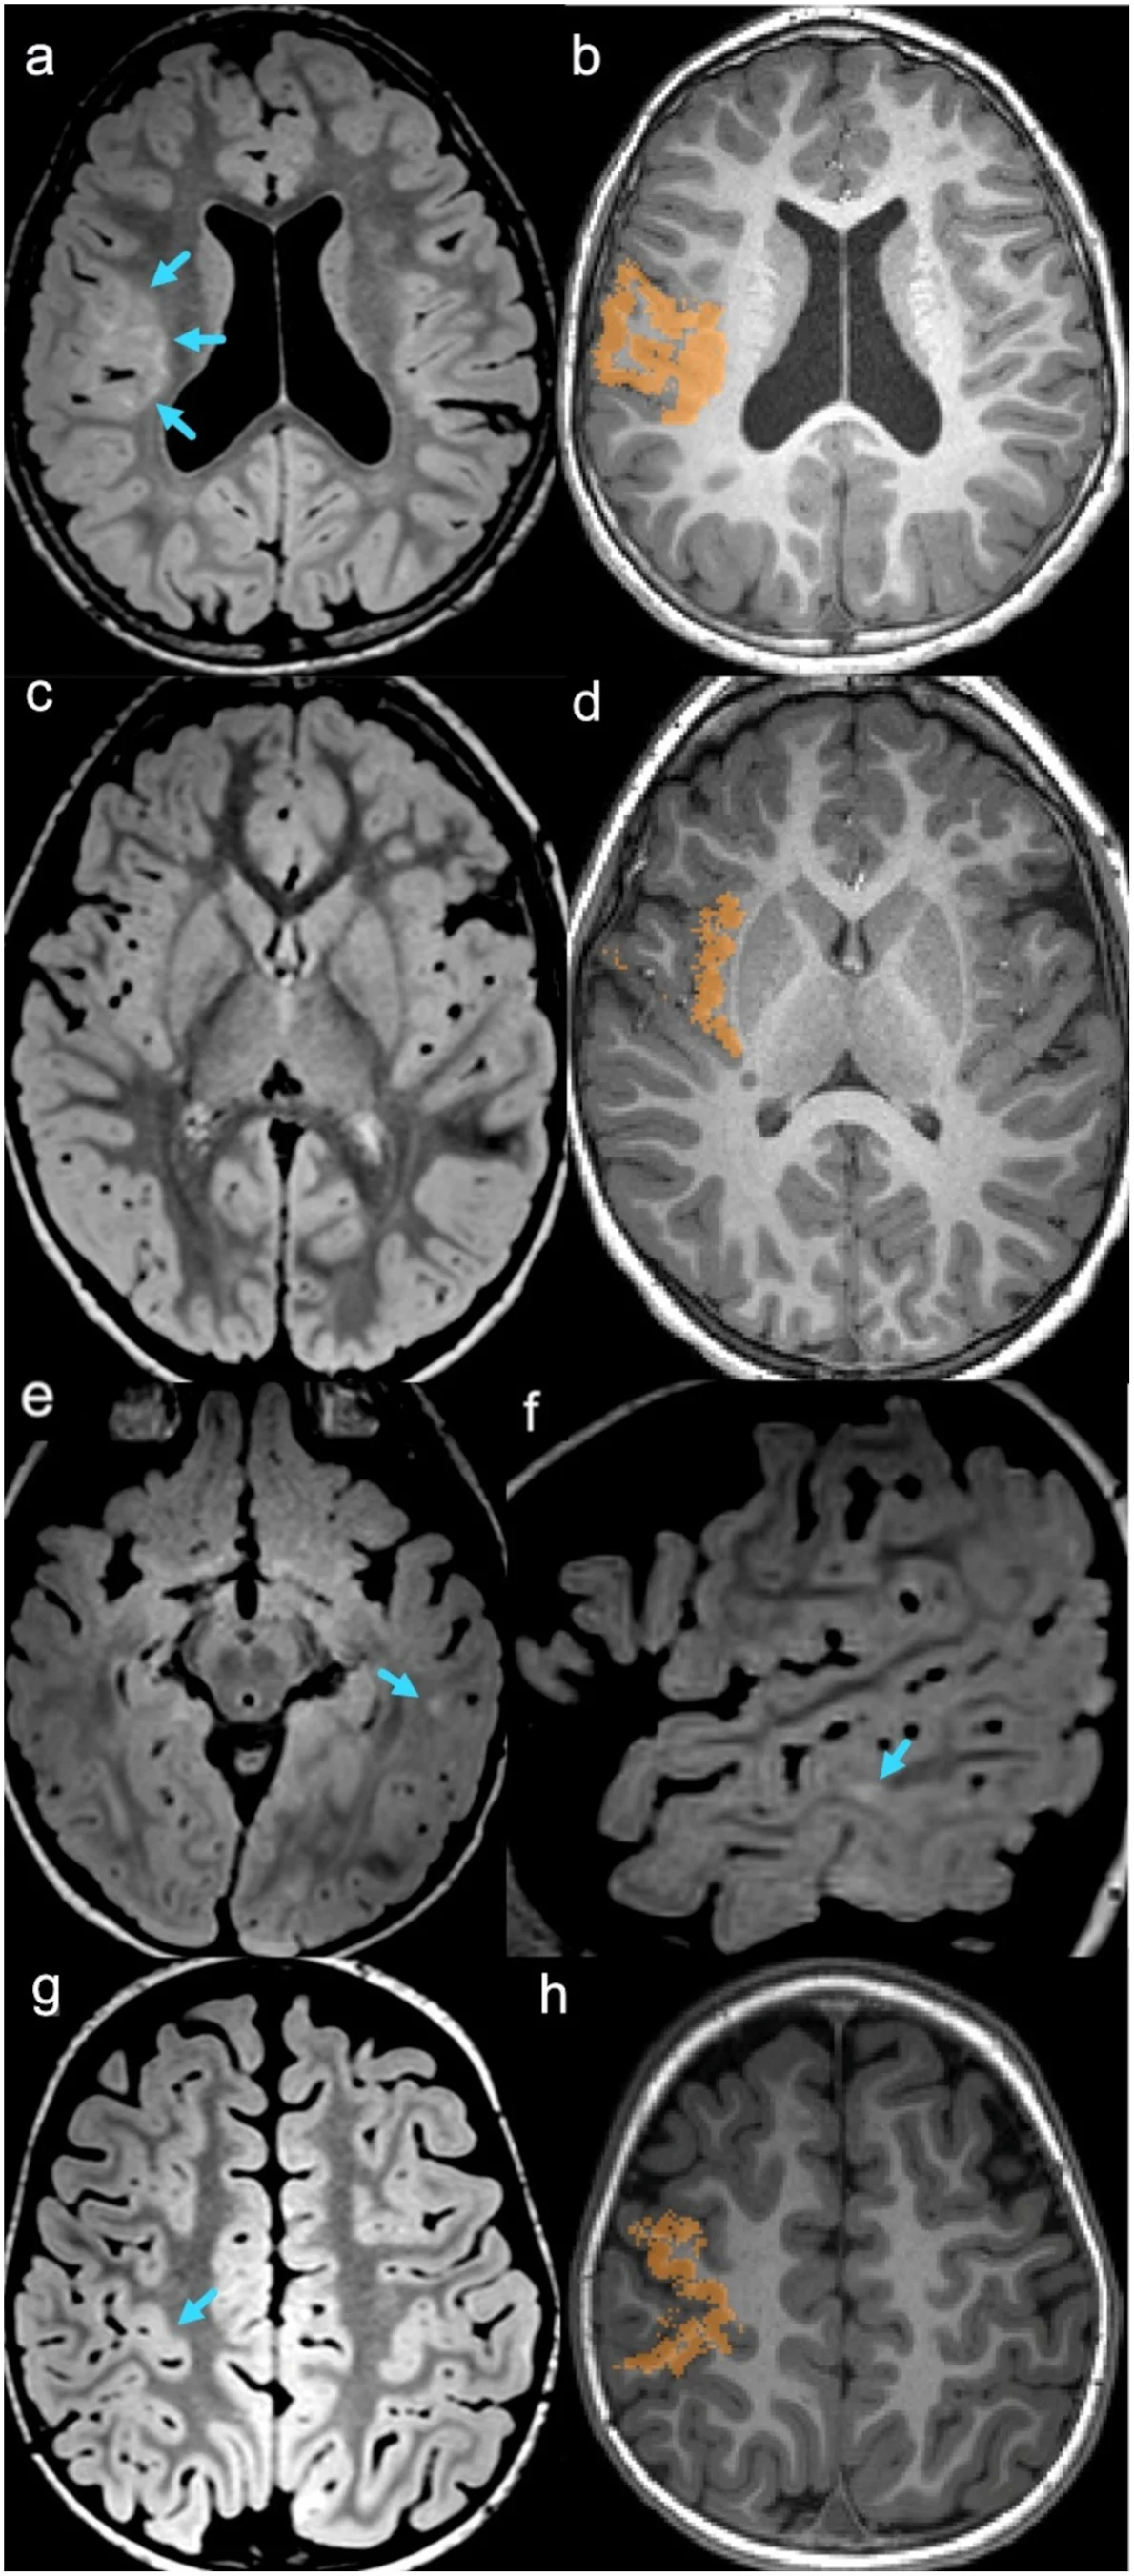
Representative imaging examples (MELD Graph): (a–b) Correctly identified lesion (TPL) in the right insula consistent with presumed FCD type 2A, shown on FLAIR (blue arrows, a) and the corresponding MELD Graph lesion mask on 3D T1-weighted MRI (b). (c–d) False-positive MELD Graph detection (FPL) (d) with the corresponding FLAIR image (c). (e–f) Lesion missed by MELD Graph (FNL), visible on FLAIR (blue arrows). (g–h) Retrospectively identified candidate lesion on FLAIR (rCL) (blue arrow, g) with the corresponding MELD Graph mask on 3D T1-weighted MRI (h).
